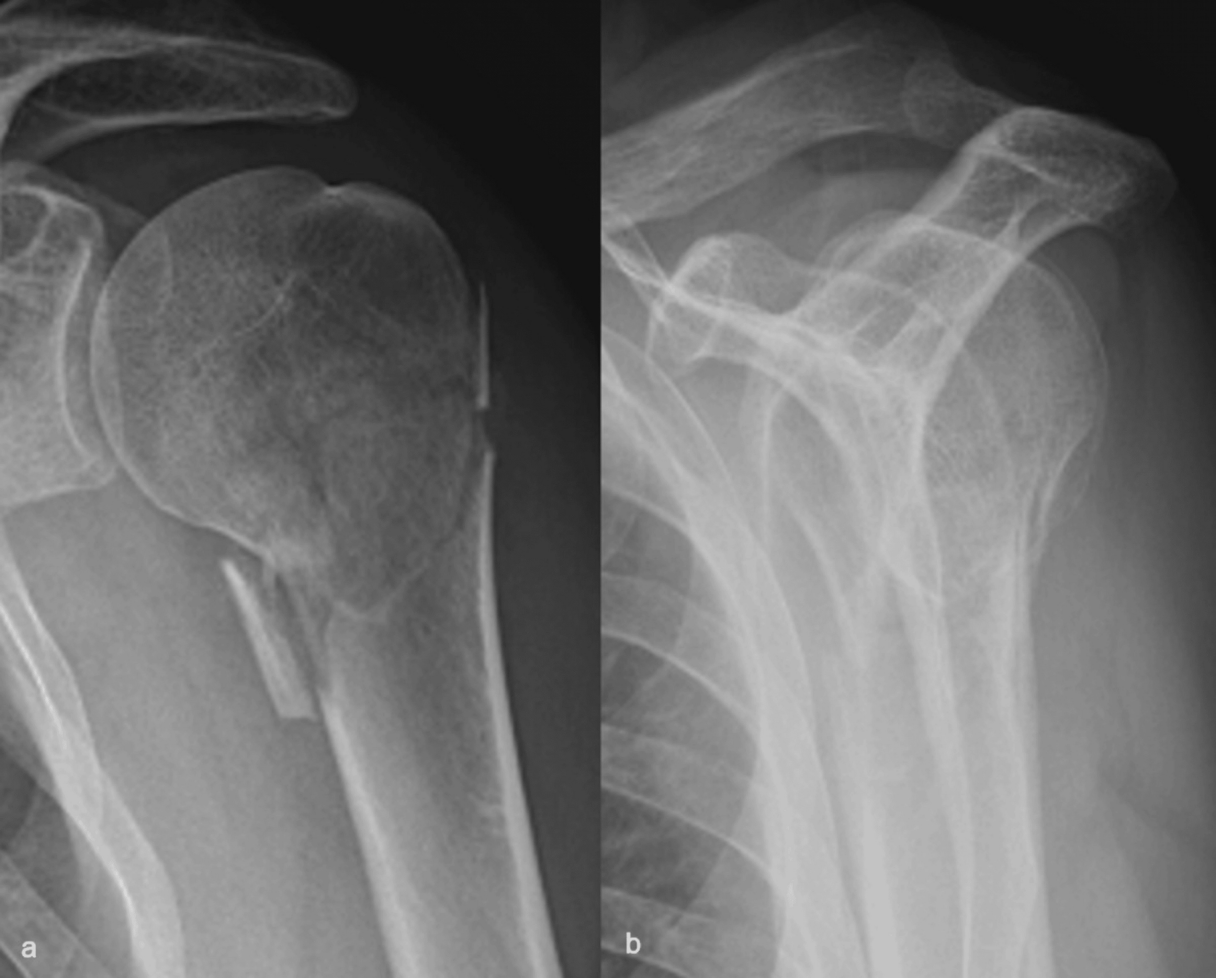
The initial fracture on AP (a) and lateral (b) X-rays: essentially undisplaced with some medial comminution
